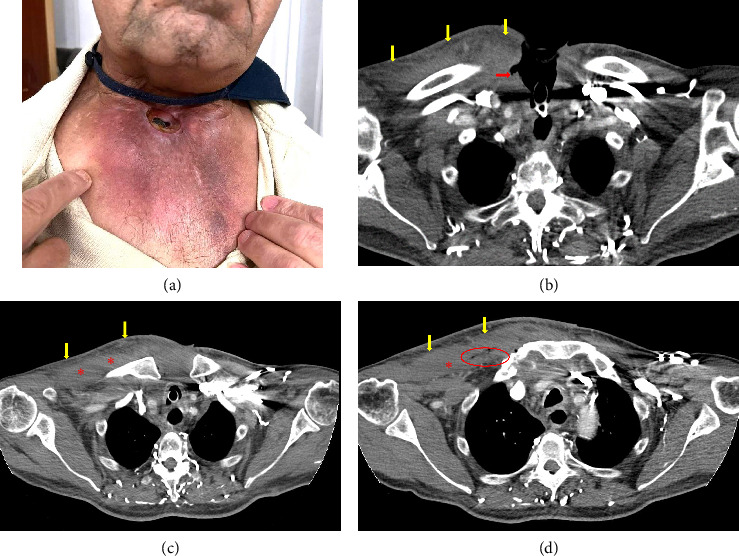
(a) Initial case presentation: right SCJ and adjacent anterior chest wall cellulitis. First axial CT scan slides (b). The red arrow shows focal ulceration of the right side of the tracheostomy; the yellow arrows show soft tissue inflammatory changes (cellulitis). (c and d) Chest wall inflammatory changes (cellulitis) and right pectoral major myositis (asterisk) with gas formation (red circle).
